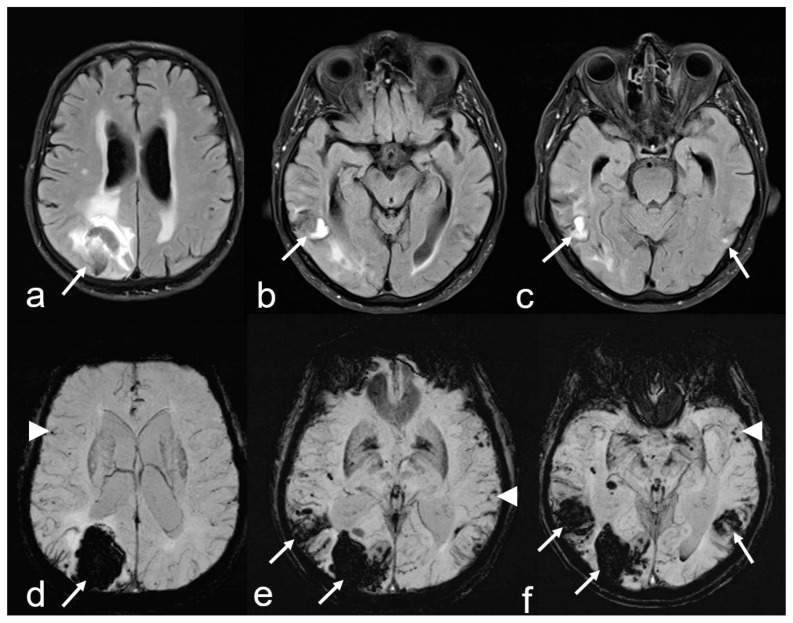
Recurrent intracerebral hemorrhages (ICH) within 2 years in a 74-year-old man with a history of progressive cognitive impairment. The patient was on antihypertensive medication and a statin but had neither antithrombotic drugs nor anticoagulant therapy. The final diagnosis was new lobar ICB due to probable CAA in accordance with the 2.0 version of the Boston criteria [38]. Axial FLAIR (fluid attenuated inversion recovery) images (a–c) showing three ICHs at different time points parieto-occipital right ((a,d): arrow), temporal right ((b–e): arrow) and temporal left ((c,f): arrow); (d–f): susceptibility-weighted imaging (SWI; arrow) disclosing additional multiple microbleeds (MB) with temporal accentuation (d–f: arrowhead); MRI 1.5 T Siemens AREA.
